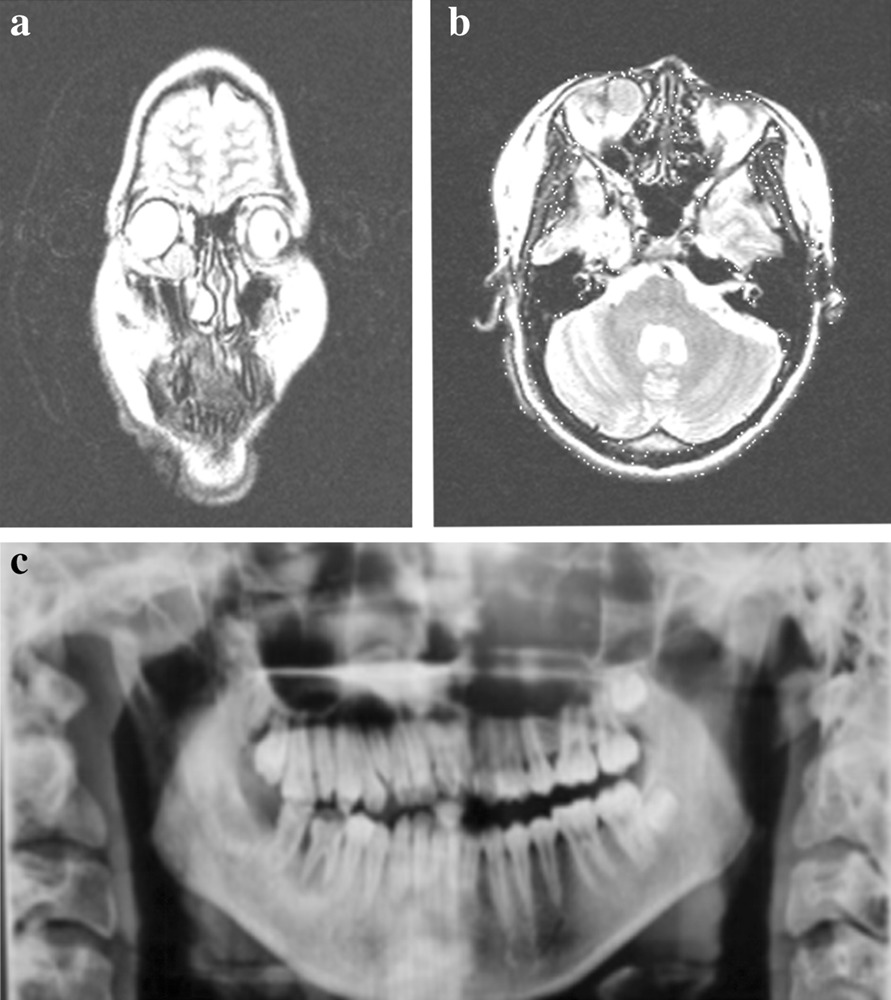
a, b Plain orbital MRI scan reveals an 18-mm diameter abnormal intensity signals in the right lacrimal sac. T1WI shows hyperintensity while T2WI shows hypointensity. Partial destruction of the right orbital bone and ethmoid bone is noticed. c Orthopantomography shows poor development of the right upper and lower jaw bones as well as alveolar bones
